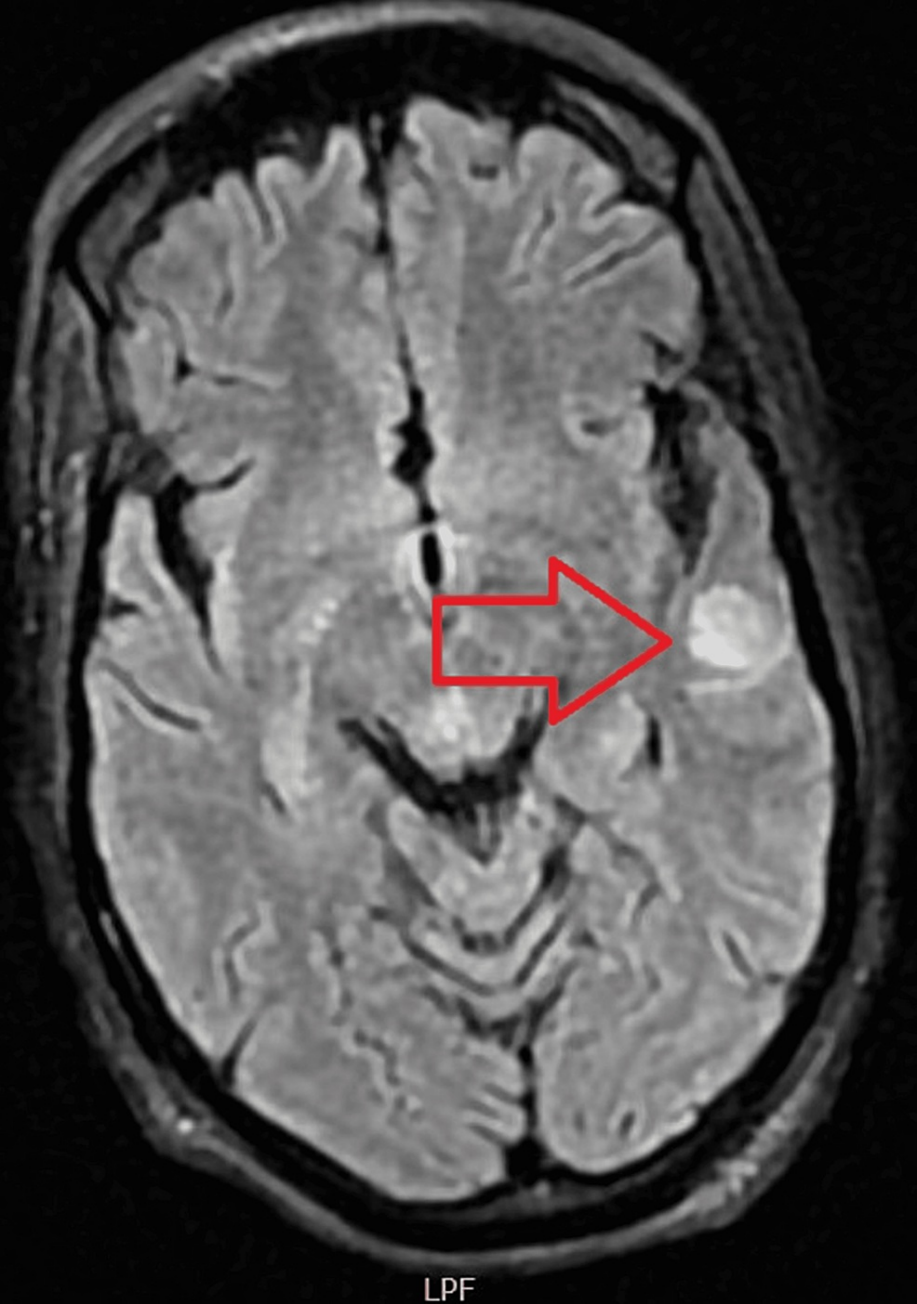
Cranial MRI showing left temporal hyperdensity in T2 sequence (red arrow).
